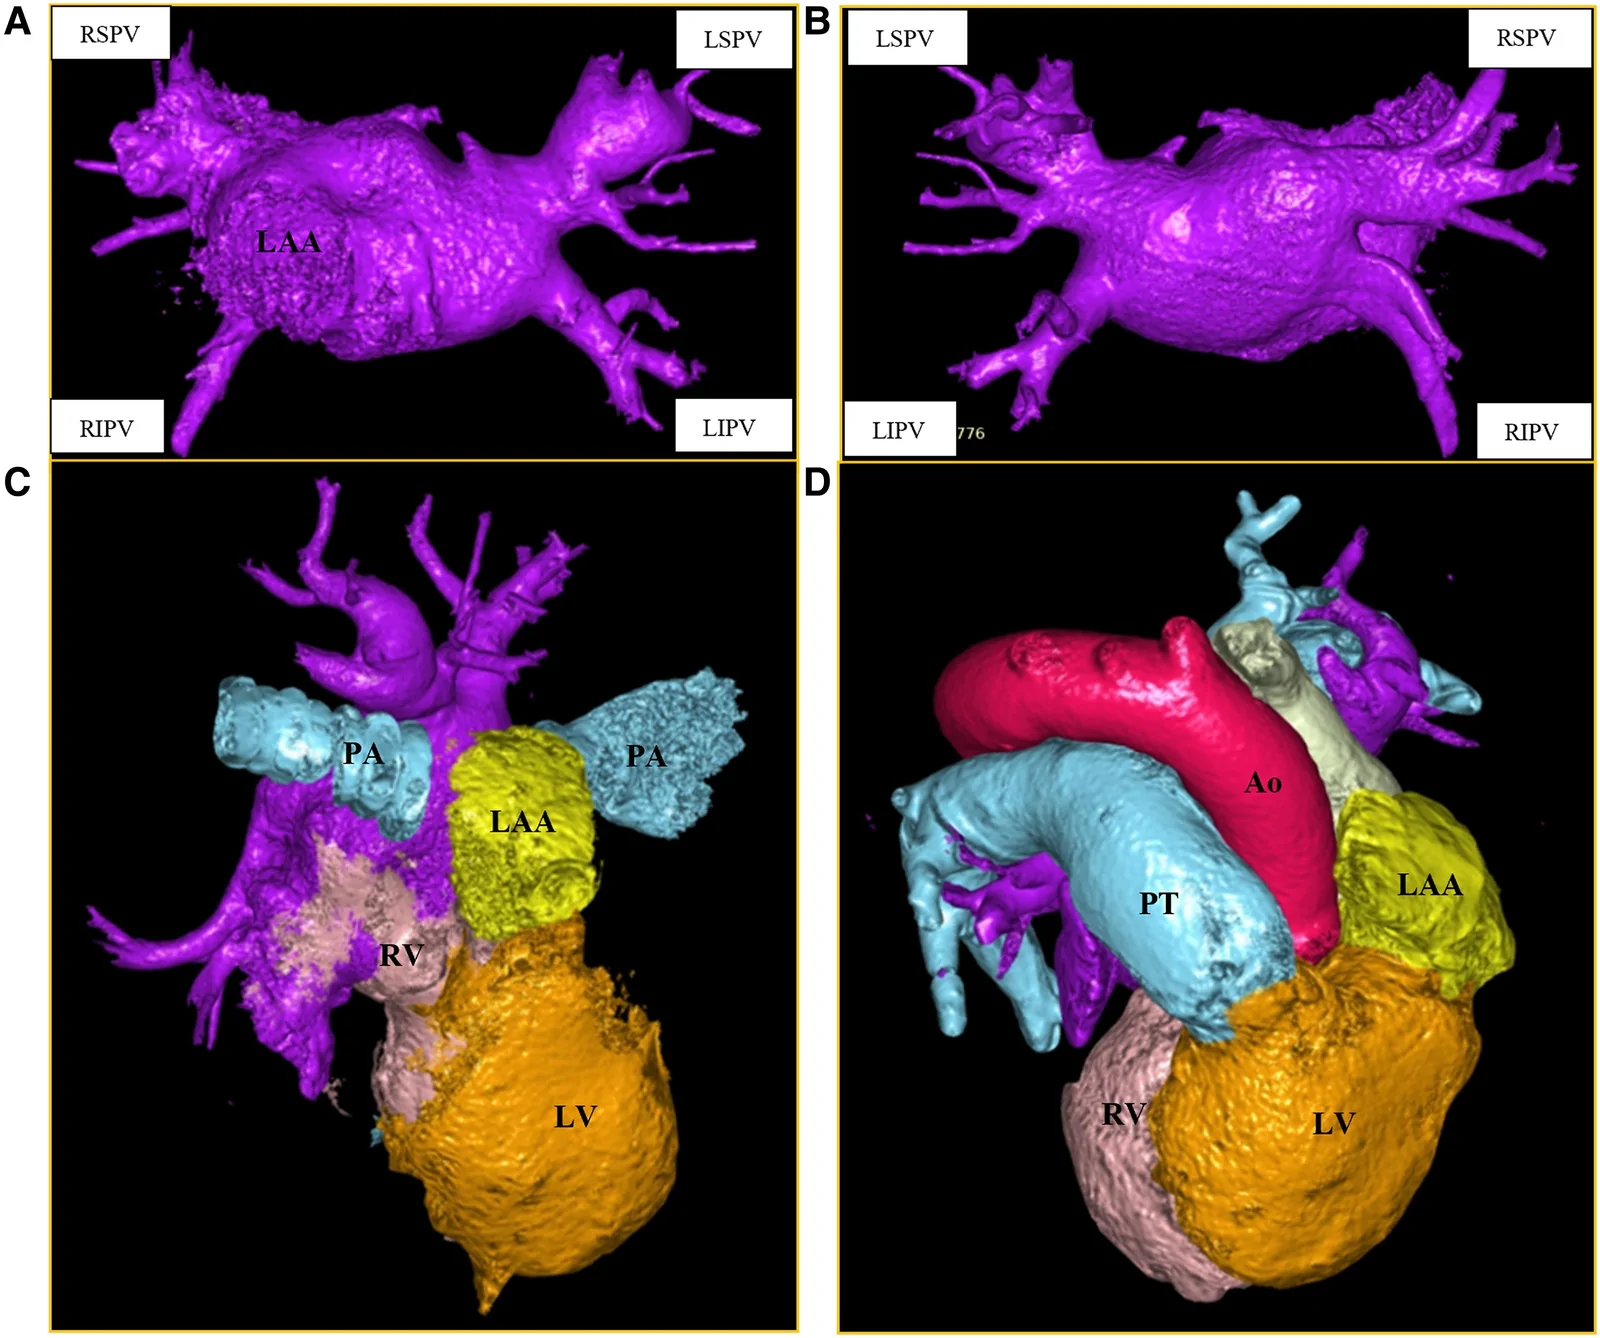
Computed tomography with 3D reconstruction. (*A*, *B*) Pulmonary veins in anterior (*A*) and posterior (*B*) views. (*C*, *D*) Heart in lateral (*C*) and anterior (*D*) views, demonstrating dextrocardia. SPV, superior pulmonary vein; IPV, inferior pulmonary vein; PA, pulmonary artery; LAA, left atrial appendage; RV, right ventricle; LV, left ventricle; PT, pulmonary trunk; Ao, Aorta.
